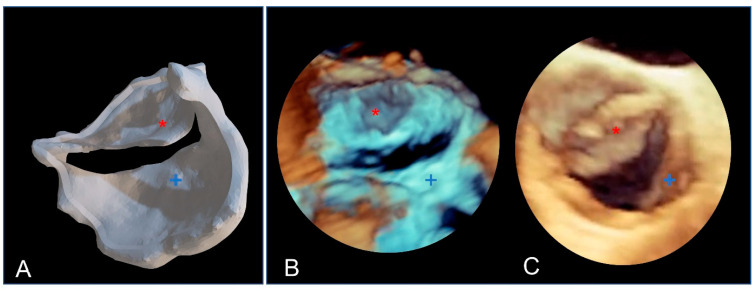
The bicuspid aortic valve in a similar face-plan view. (A) View of the 3D printed model. (B) A 3D ultrasound view of the immersed model (with two thin aortic cusps marked with * and +). (C) A 3D ultrasound in situ view of the same valve in the patient, showing both cusps (+ and *).
